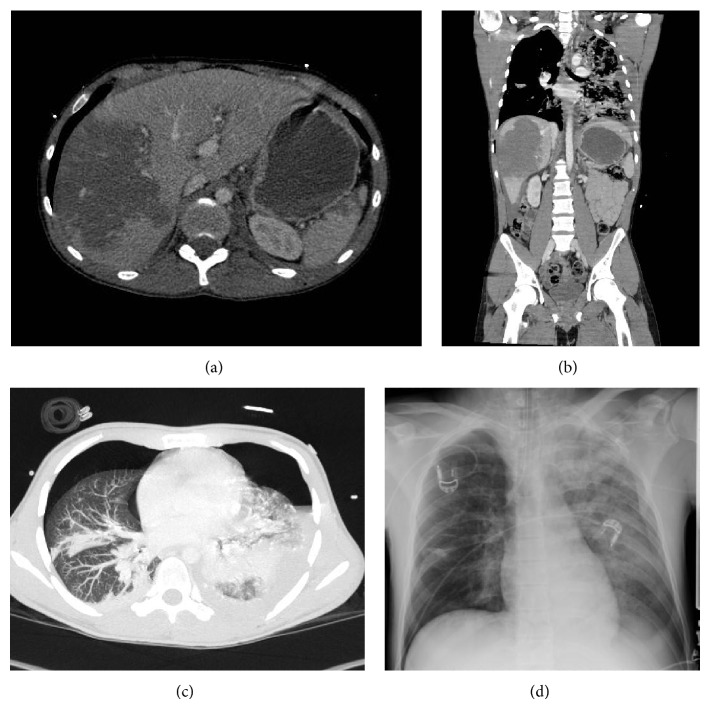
Radiologic evaluation of the intra-abdominal injuries: (a) and (b) imaging of the liver and spleen laceration with consecutive intra-abdominal hematoma in horizontal and frontal planes; (c) and (d) imaging of the hematothorax on the left side and pneumothorax on the right side in the horizontal plane and in conventional radiographs.
